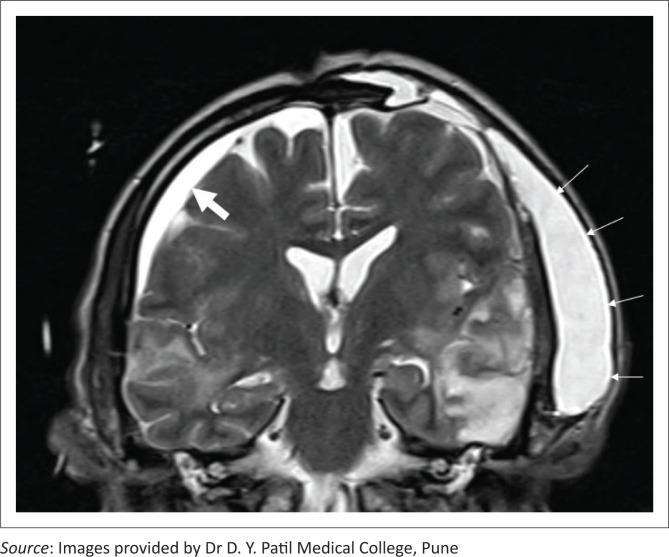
Subgaleal and subdural hygromas. Coronal T2 weighted MRI in a patient with a left parietal craniectomy shows a hyperintense fluid collection in the left subgaleal region, extending beyond the margins of the craniectomy to the left temporal region (thin white arrows). An extra-axial subdural collection is also noted in the right frontoparietal region (thick white arrow).
